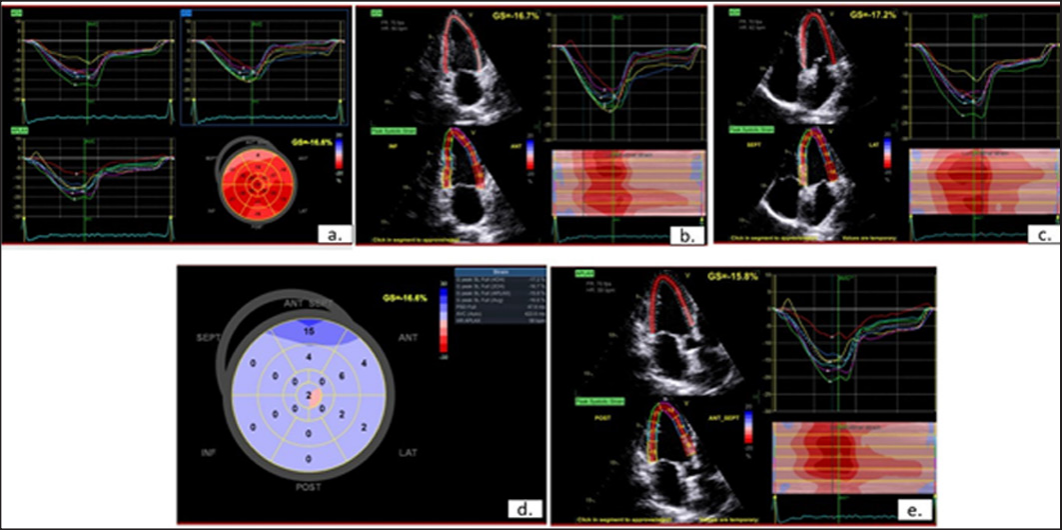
Speckle tracking echocardiogram measuring global longitudinal strain (a, b, c, e) and post systolic shortening (d).
